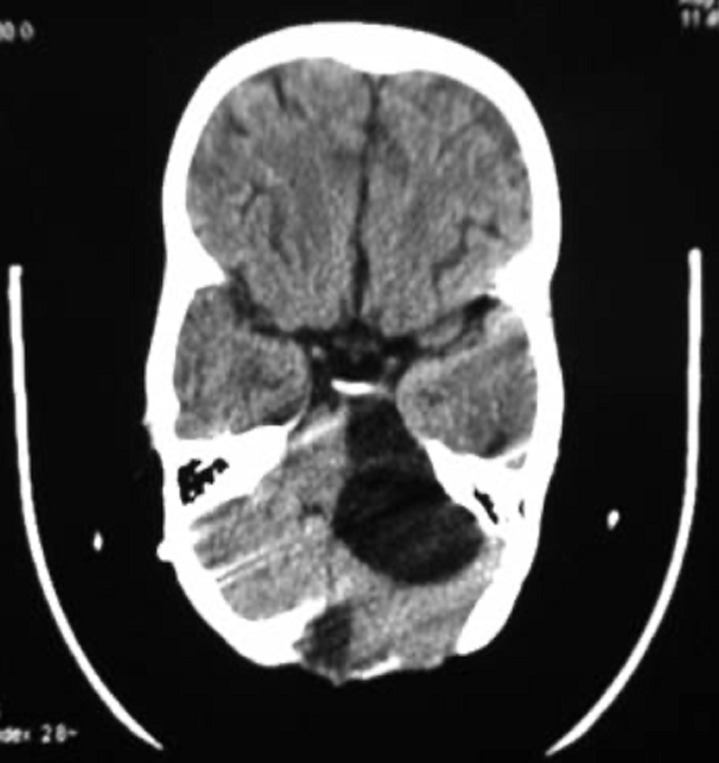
postoperative cerebral CT scan which allows to visualizing the total excision of the dermoid cyst via the median sub-occipital approach, as well as the arachnoid cyst of the cerebellopontine angle not yet operated
